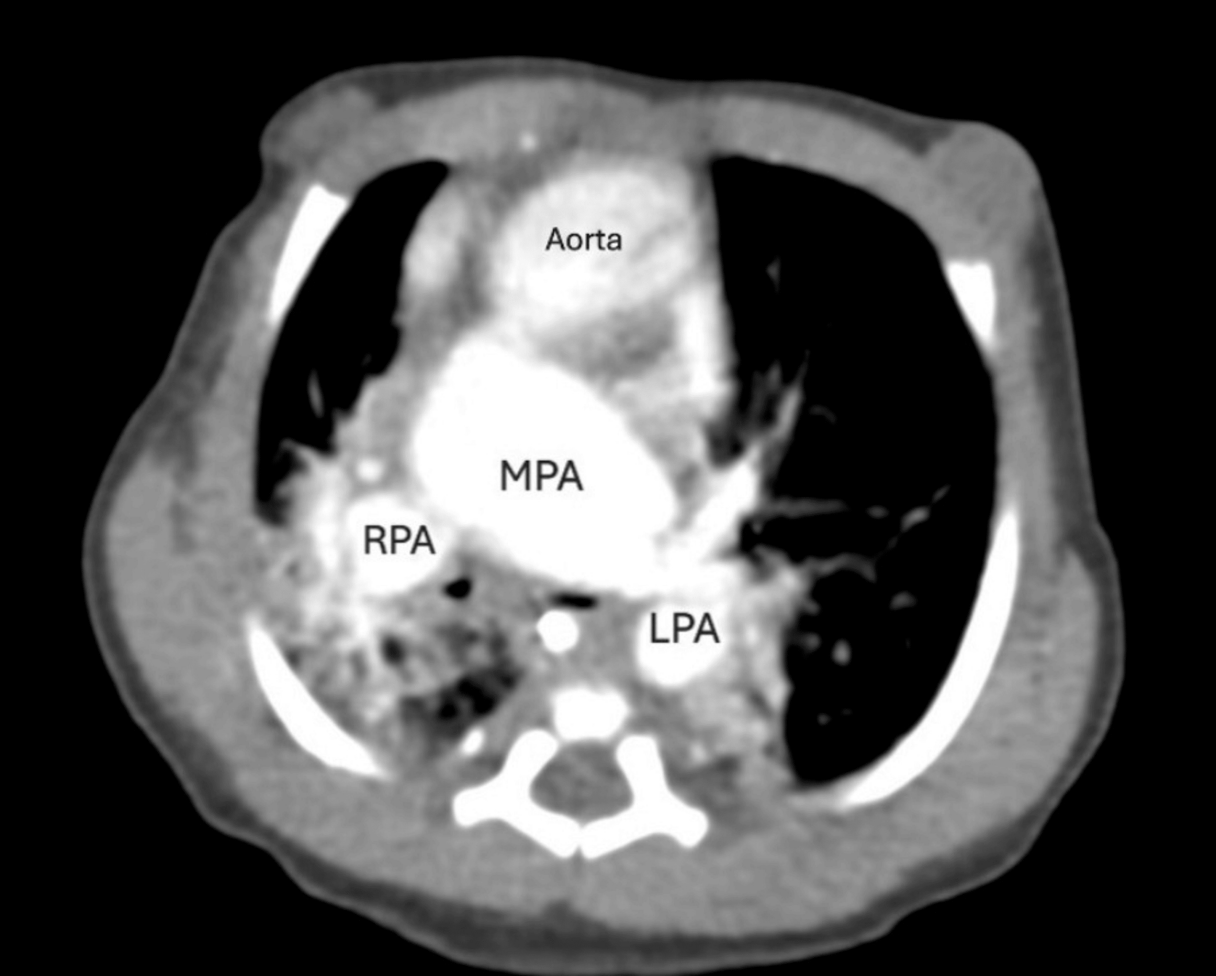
Axial contrast-enhanced CT angiography demonstrates a dilated main pulmonary artery (MPA) with clear bifurcation into the right pulmonary artery (RPA) and left pulmonary artery (LPA). Aorta is anterior to the MPA.
